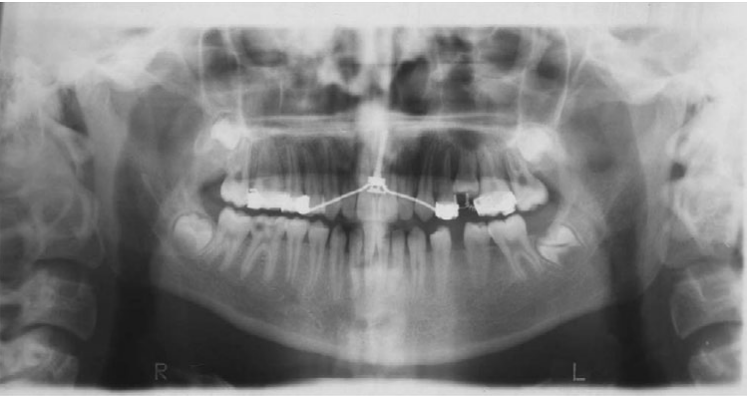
After distalization panoramic radiograph of the patient.
